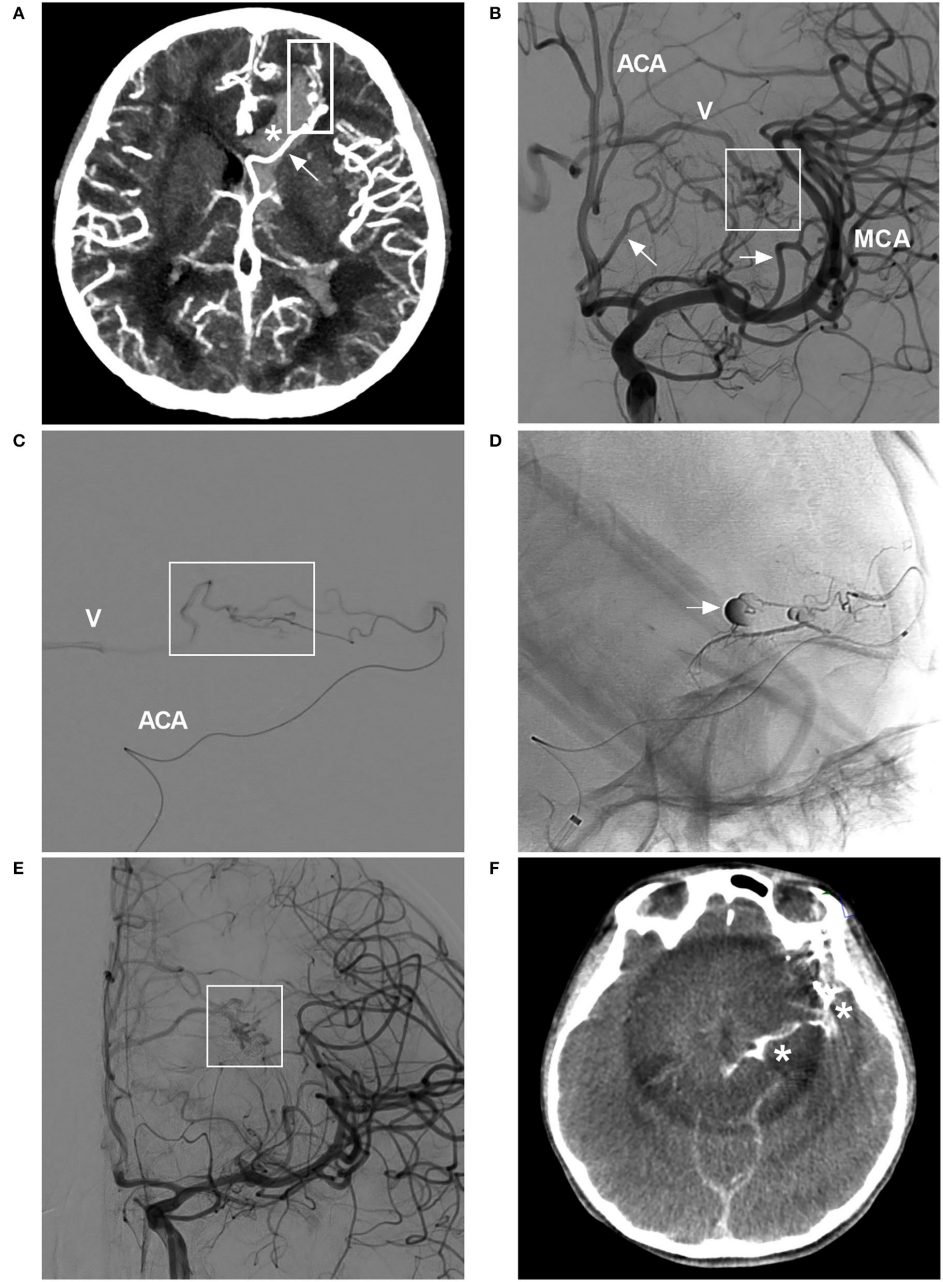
BAVM with intraoperative rupture from Onyx casting (case 25). (A) CT angiogram showing BAVM (frame) draining into the deep vein system (arrow), with a rupture into the ventricle (asterisk). (B) Angiogram of the ICA showing the BAVM (frame) supplied by the ACA and MCA (arrows), with drainage into the deep vein system (V). (C) Selective microcatheter angiogram of the branch of the ACA showing part of the nidus (frame) and draining vein (V). (D) Unsubtracted angiogram showing rupture of the nidus during Onyx casting (arrow). (E) Angiogram of the ICA showing less residual BAVM nidus (frame) after Onyx casting *via* the ACA and MCA. (F) X-per CT showing contrast agent extravasation. ACA, anterior cerebral artery; BAVM, brain arteriovenous malformation; CT, computed tomography; ICA, internal carotid artery; MCA, middle cerebral artery.
